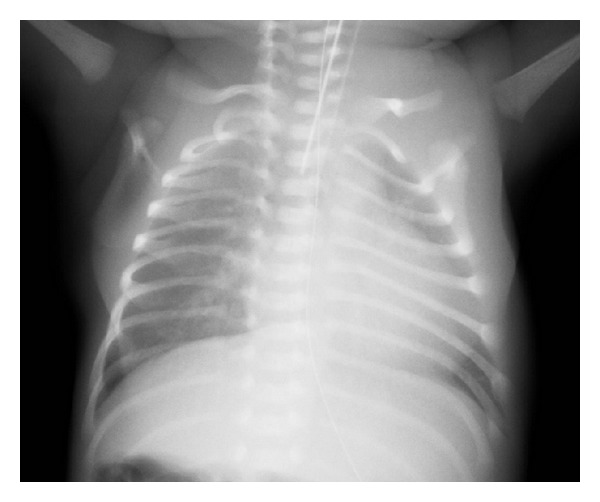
The chest X-ray of the first recipient-twin showed cardiac dilatation: the cardiothoracic ratio was 70%.
